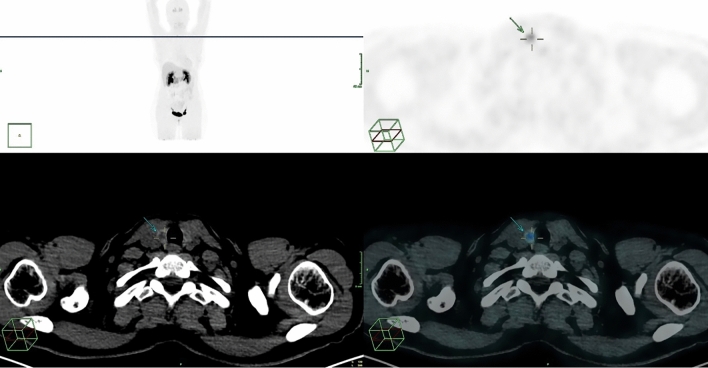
[^18^F]FDOPA PET/CT preoperative staging in a 61 y.o. woman with hypercalcitonemia and mild elevation of serum CEA, showing focal uptake in thyroid nodule in the right lobe; no evidence of pathological lymph nodes. A MTC was diagnosed after total thyroidectomy
